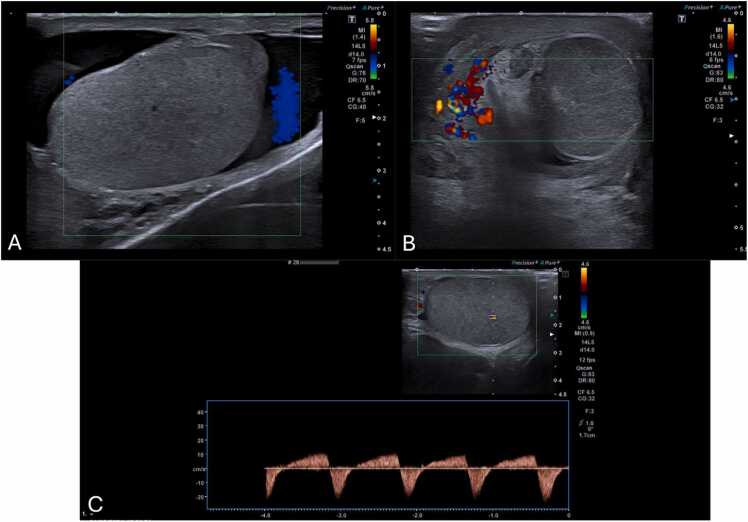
(A–C) Scrotal ultrasound in different male adolescent patients with surgically confirmed testicular torsions. (A and B) Sagittal and transverse color-Doppler US of torsed testes, with loss of normal blood flow on color-Doppler, depicting the typical ultrasonographic appearance of this condition. Notice that the parenchyma has as homogenous echotexture, indicating that the torsion is recent and the testis salvageable. (C) Sagittal testicular Spectral Doppler-US in a 16-year-old presenting with reduced testicular parenchymal color Doppler signal as well as abnormal high resistive blood flow (RI > 0,75), indicating the presence of obstruction to the normal blood flow.
